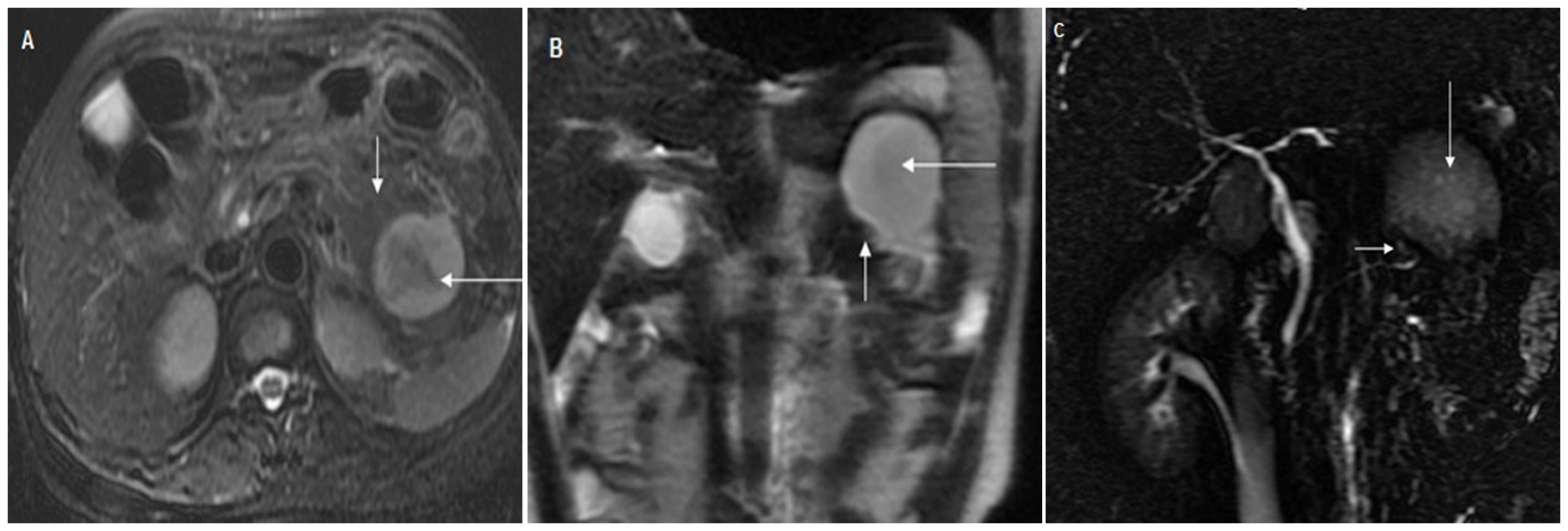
Pancreatic duct disruption in a 46-year-old female with acute pancreatitis. The Acute Physiology And Chronic Healthy Evaluation II score was 4, and the magnetic resonance severity index was 8. (A) Axial fast-recovery fast spin-echo T2-weighted image with fat suppression, (B) coronal single-shot fast spin-echo T2-weighted image and (C) magnetic resonance cholangiopancreatography show a 5-cm-diameter ﬂuid collection replacing the tail of the pancreas (long arrow). The main pancreatic duct (short arrow) enters the fluid collection.
